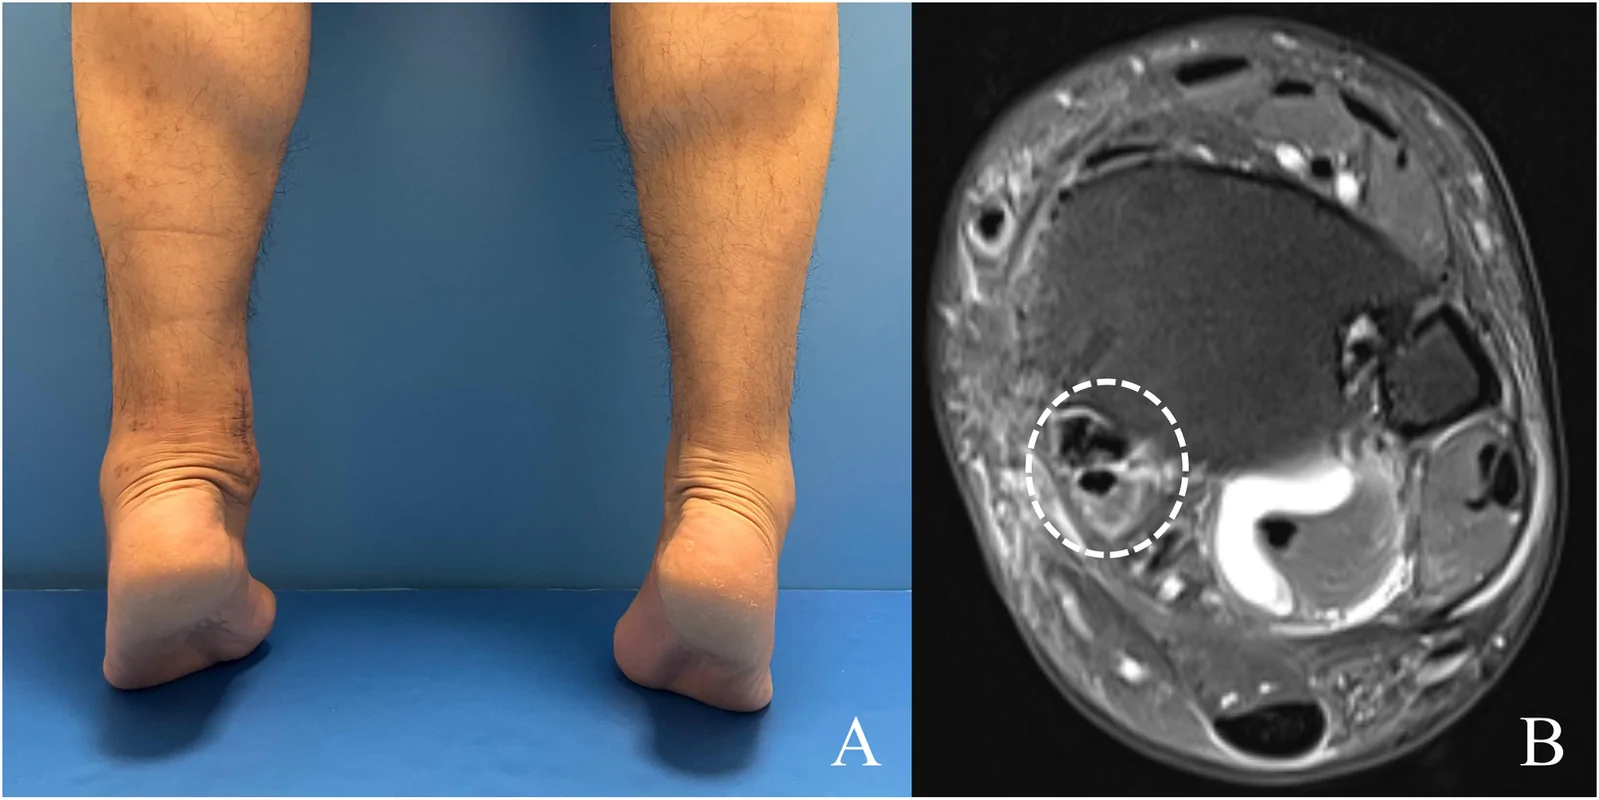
One-year postoperative follow-up. (A) Successful completion of the single-limb heel rise test with restoration of hindfoot inversion. (B) Follow-up MRI of the ankle showing reduced peritendinous fluid accumulation around the posterior tibial tendon sheath compared to preoperative imaging (dashed circle), consistent with satisfactory surgical outcomes.
